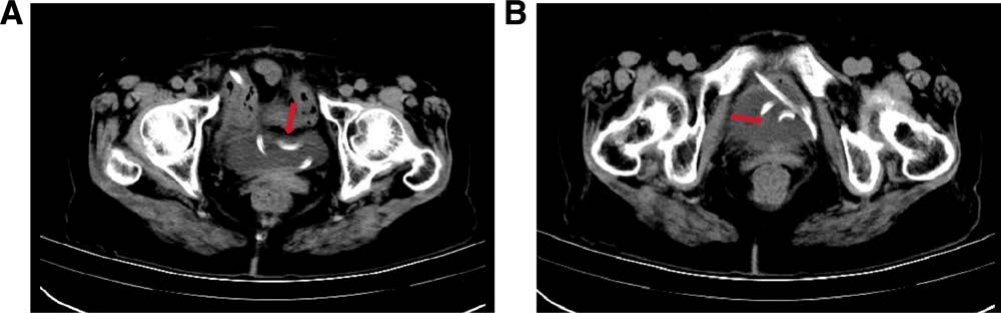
(A and B) The CT scan suggests that the PD catheter has penetrated the bladder. CT = computed tomography, PD = peritoneal dialysis.
